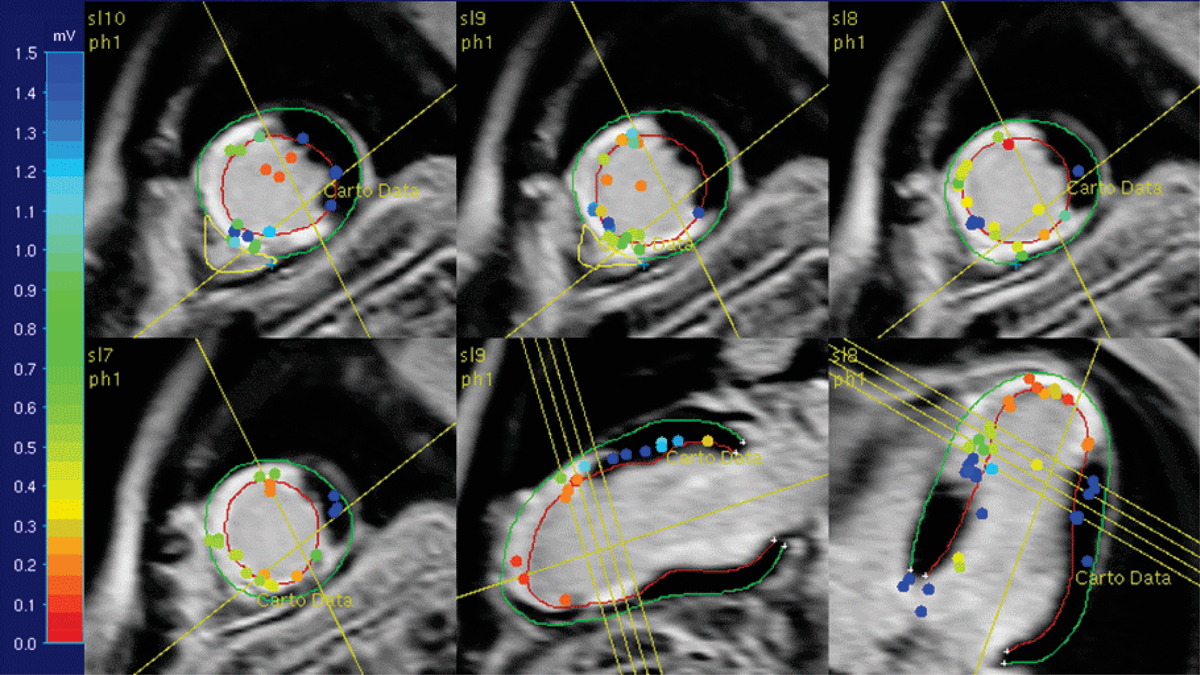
Late enhancement MRI of the same patient showing transmural scar in the septal-apical region with results of EAM superimposed. Bipolar voltages are low (<1.5 mV) in the scar region and normal (>1.5 mV) in the healthy myocardium.
